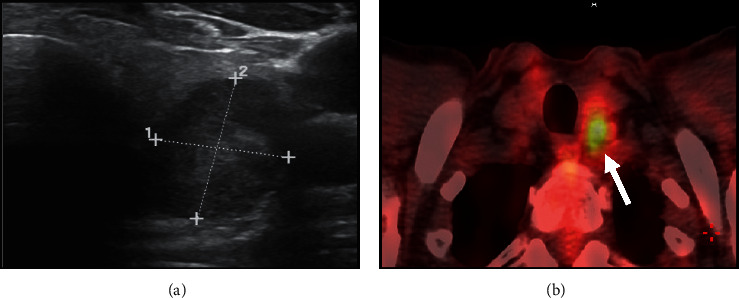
Ultrasonographic suspicious features of the large solid hypoechoic and taller-than-wide shape thyroid nodule located in the mid-inferior part of the left lobe (a), which showed significant tracer uptake at 18F-FDG PET/CT (arrow, (b)).
